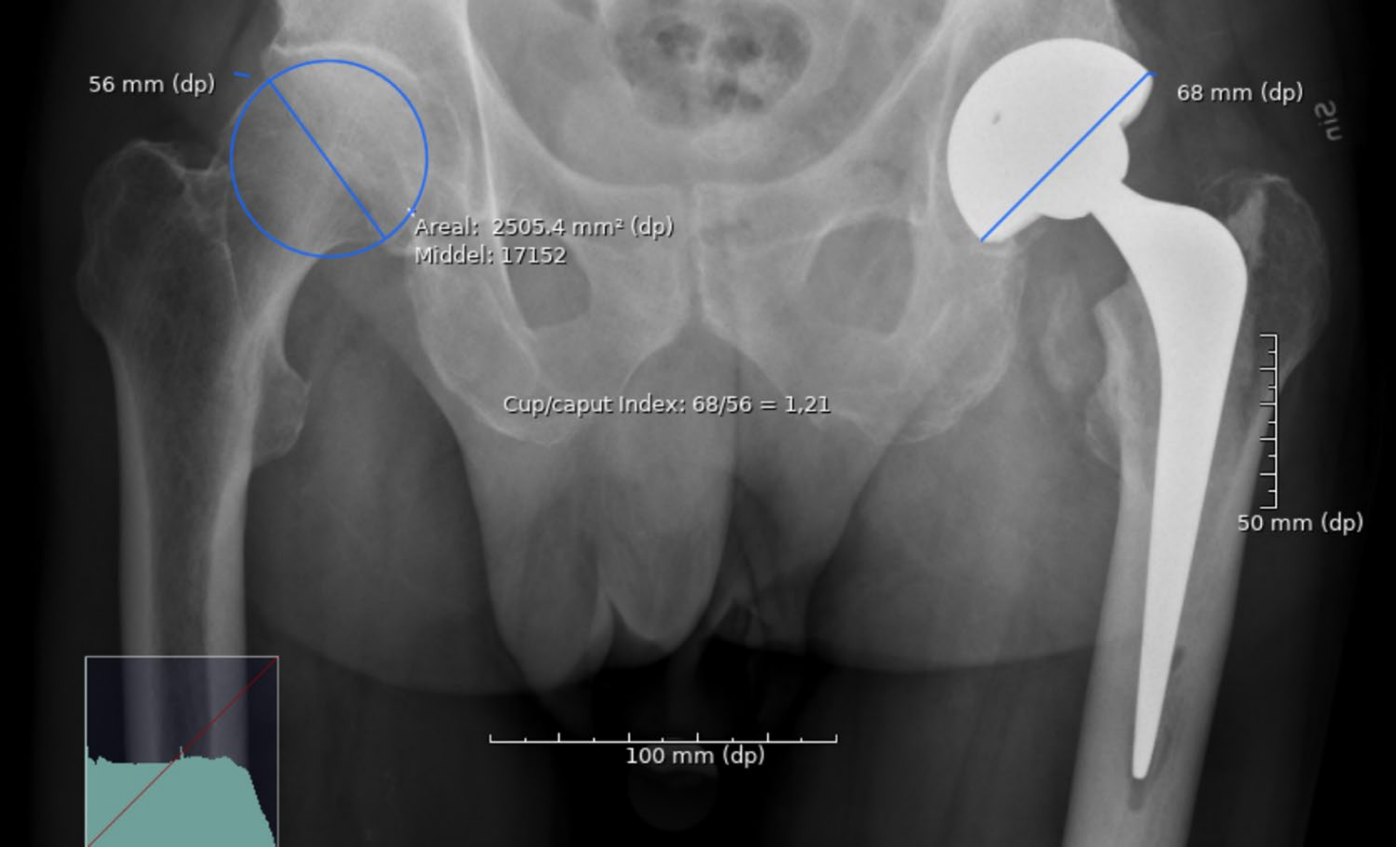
Cup/caput Index measured on AP pelvic radiograph
